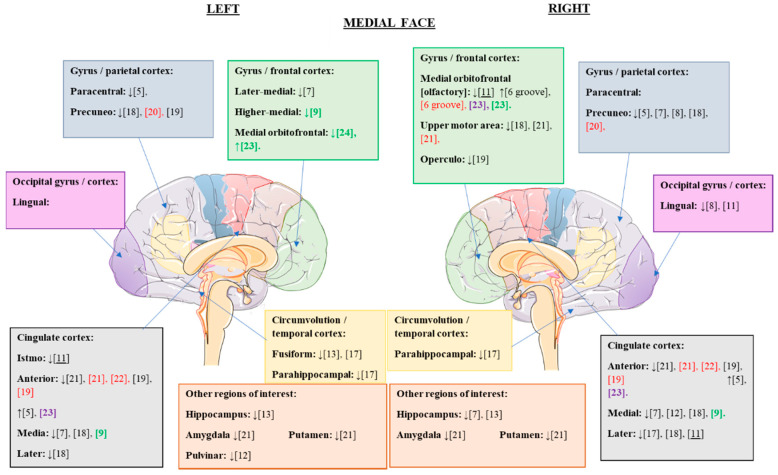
Cortical structural alterations in GMV and CT that were statistically significant in the different eating disorders compared to the control values; medial view. ↑, increased GMV/CT; ↓, reduced GMV/CT; citations in black, changes observed in patients with AN versus controls; citations in red, changes observed in patients with REC versus controls (possible trait and nonstate changes); citations in green, changes observed in patients with BN versus controls; underlined citations, changes observed in patients with AN versus REC; citations in purple, changes observed in patients with binge-eating disorder versus controls.
